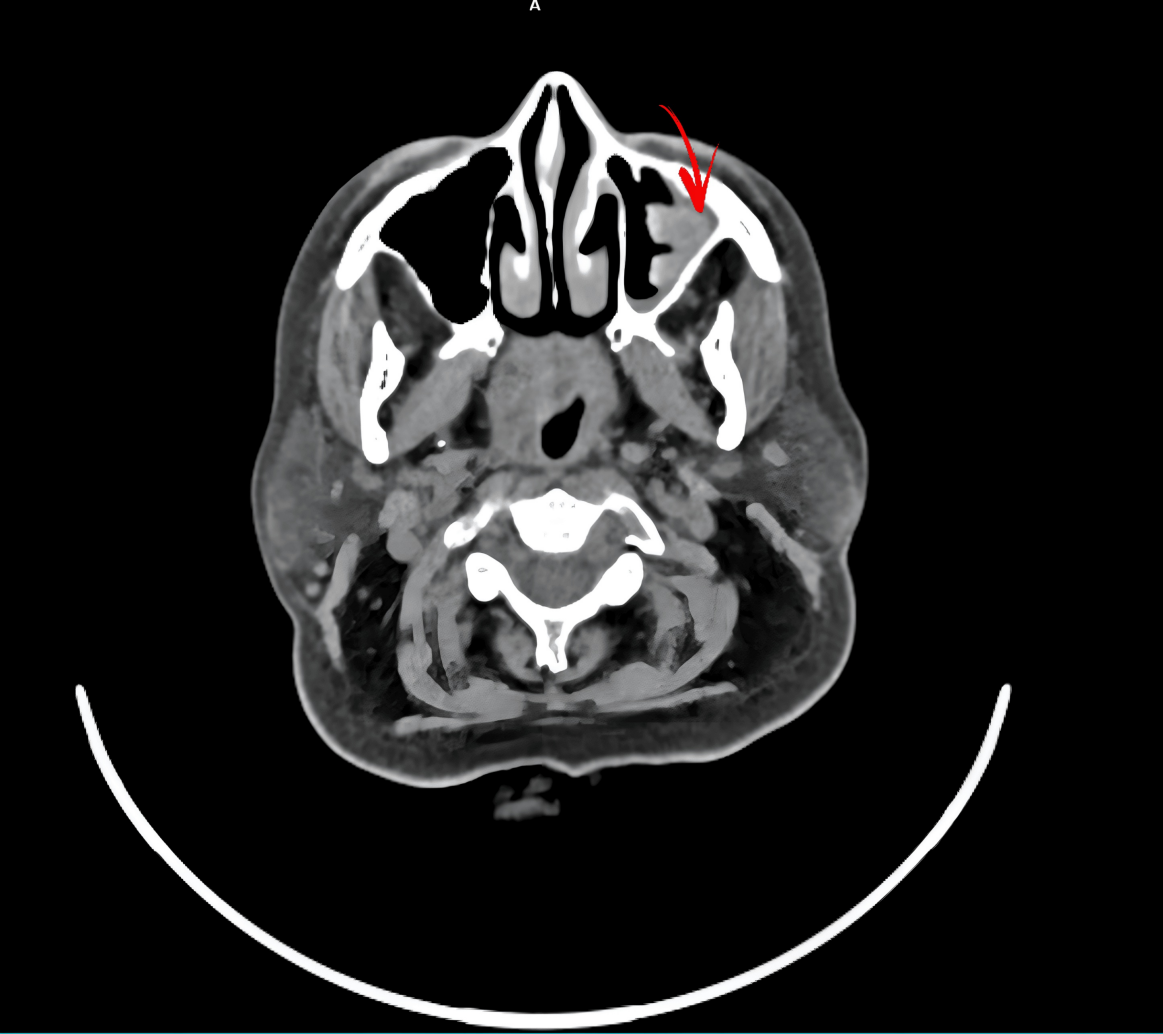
CT of the paranasal sinuses and base of the skull. CT shows moderate irregular mucosal thickening in the left maxillary sinus and mild paucity of right mastoid air cells.
